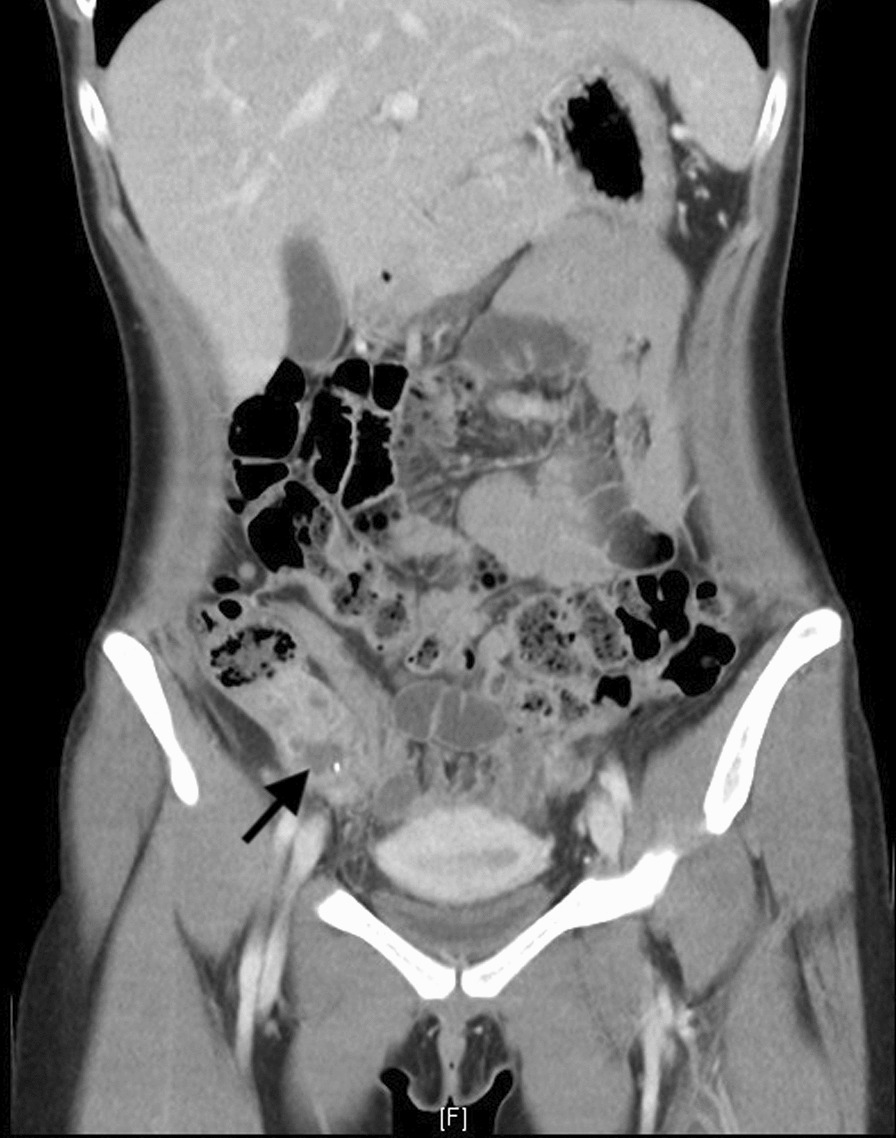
Initial contrast-enhanced abdominal CT revealed an engorged appendix with wall thickening, appendicolith and surrounding tumor formation, compatible with ruptured appendicitis with local abscess formation. (Arrow: Appendicolith with local abscess formation)
